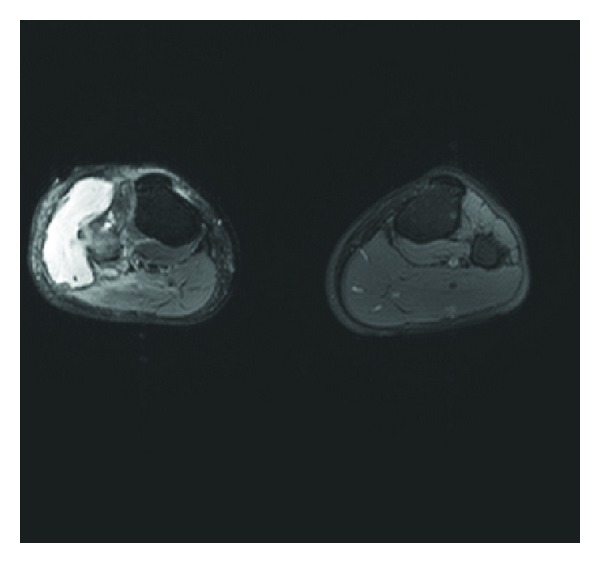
Sagittal MRI section of the lower limbs. We notice the presence of a T2 hypersignal at the level of the proximal right fibular head, with erosion of the lateral cortical bone and extension into adjacent soft tissues in the form of a collection.
